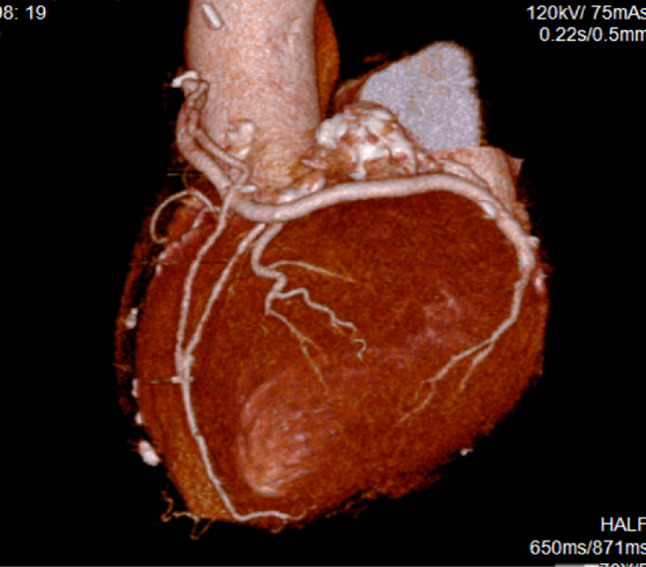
Postoperative three-dimensional computed tomography showing the final graft configuration: saphenous vein graft with piggyback anastomosis to the gastroepiploic artery supplying the left anterior descending artery, with sequential anastomoses to the first diagonal branch and the obtuse marginal artery
